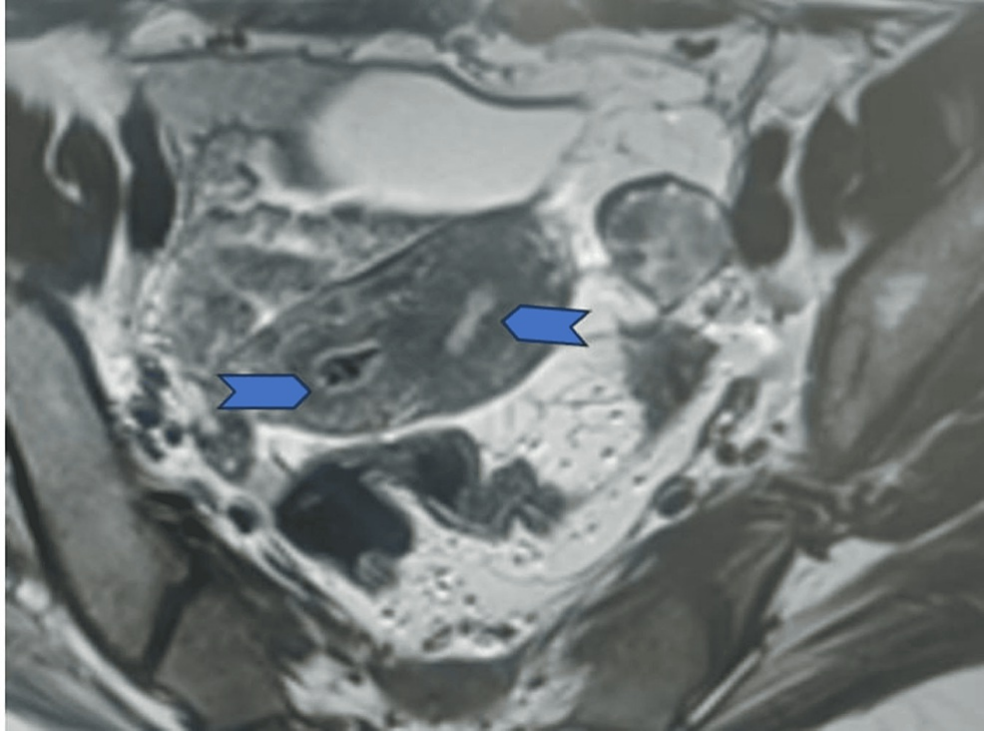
Transverse MRI section depicting double uterine cavity with intervening tissue (arrowhead: double uterine cavity)
